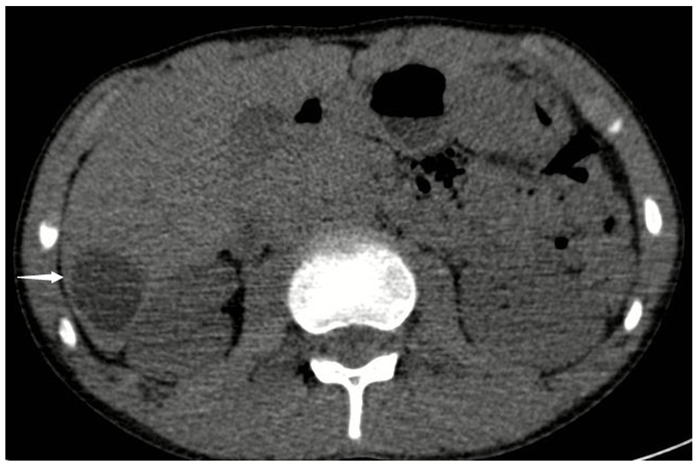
Axial computed tomography. Cystic lesion in liver segment 6 (arrow).
